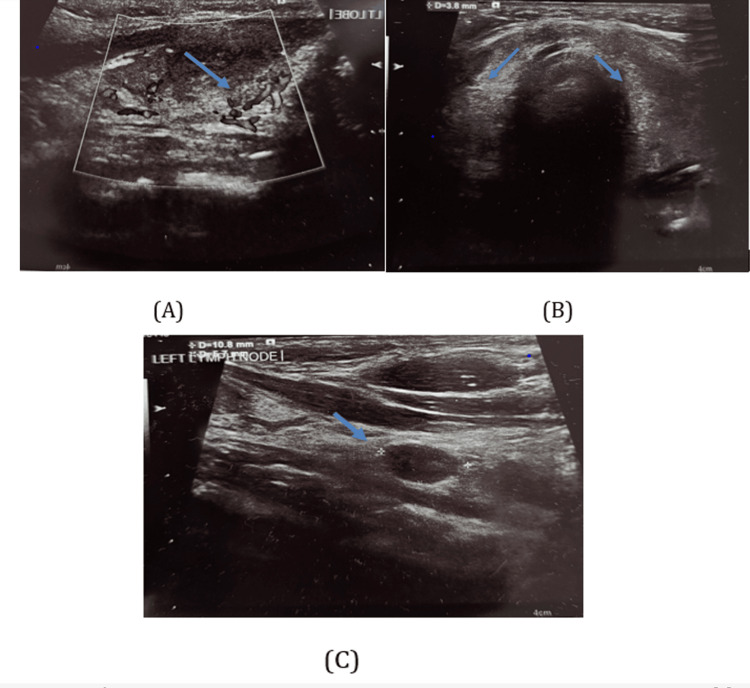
Thyroid ultrasound findings consistent with thyroiditis. (A) Increased vascularity of the thyroid gland. (B) Heterogeneous echotexture with bilateral bulky thyroid lobes and isthmus. (C) Multiple hypoechoic pre- and paratracheal lymph nodes, suggestive of reactive or inflammatory changes
